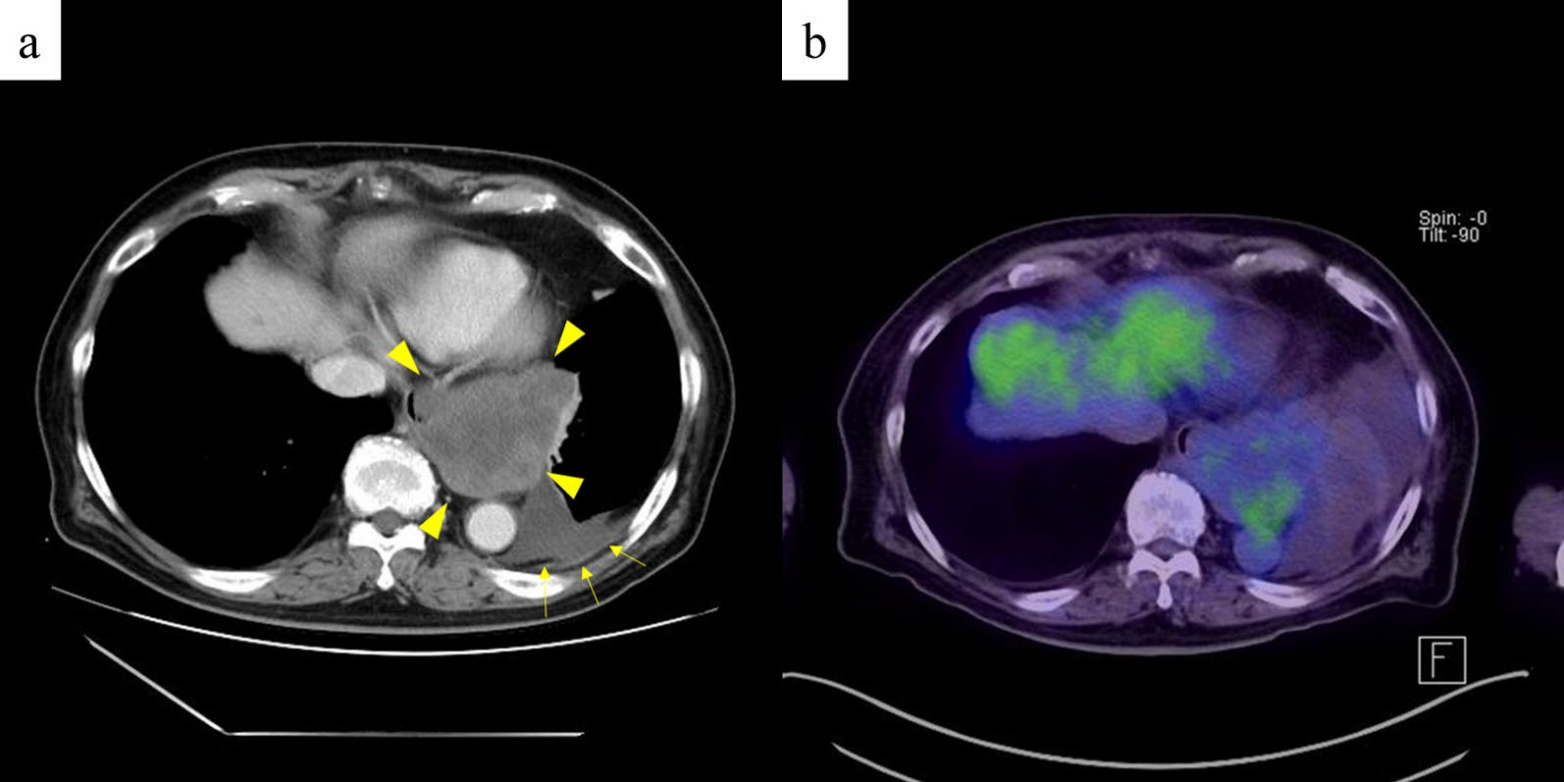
CT after neoadjuvant therapy with imatinib shows that the tumor is approximately 70 mm in diameter with pleural effusion (a) and PET–CT shows that SUV max was 2.6 with no apparant distant metastasis (b)
